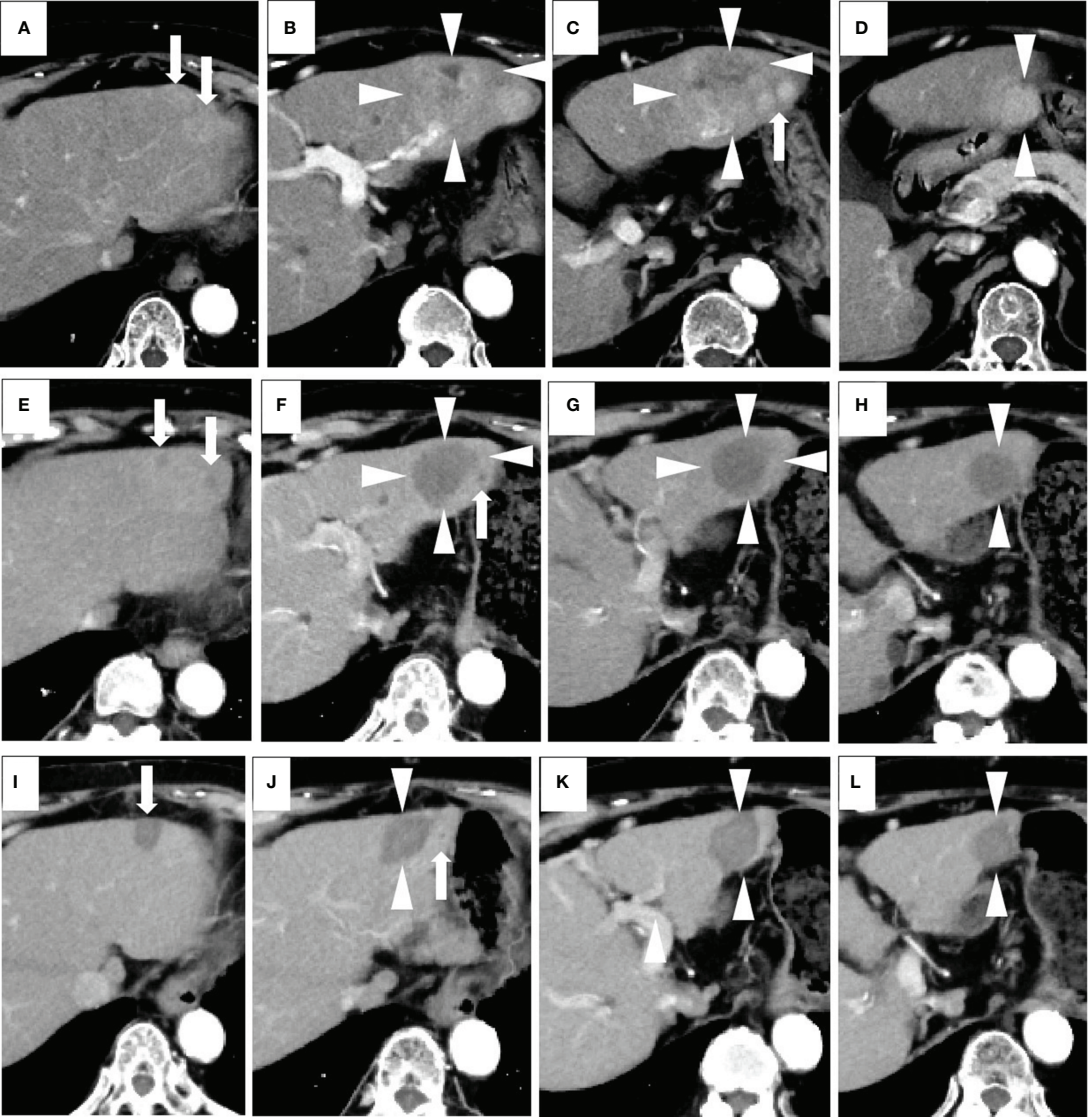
A case of combined lenvatinib and radiofrequency ablation (RFA) therapy. The patient was an 80-year-old female with no history of viral hepatitis. Her up-to-seven score was 9.3, and her Child-Pugh score was A5. All of these images were taken *via* arterial phase of contrast-enhanced CT (CECT). Images (A–D) were taken prior to the start of lenvatinib therapy. The target lesions were a larger one (a maximum diameter of 53 mm) (arrowheads) and a surrounding smaller one (arrows) located in the left lobe of the liver. Both of the tumors appeared as high-density areas. Images in (E–H) were taken 2 months after the administration of the recommended dose of lenvatinib. A marked decrease in the vascularity and size of each lesion were revealed. No newly developed lesions were observed. Therefore, this patient was evaluated as having a partial response according to the modified Response Evaluation Criteria in Solid Tumors (mRECIST). The maximum diameter of the main tumor decreased from 53 to 38 mm (arrowheads). One month after RFA, none of the lesions had any vascularity, and no new lesions were detected. Therefore, this patient was evaluated as having a complete response according to the mRECIST (image not shown). Images in (I–L) were taken 7 months after RFA (corresponding to 10 months from the start of lenvatinib), and all HCC lesions appeared as non-enhanced areas and showed a continual and marked decrease in size. Therefore, a complete response was recorded. For this patient, the initial tumor response was partial response (PR), while the best tumor response was complete response (CR).
